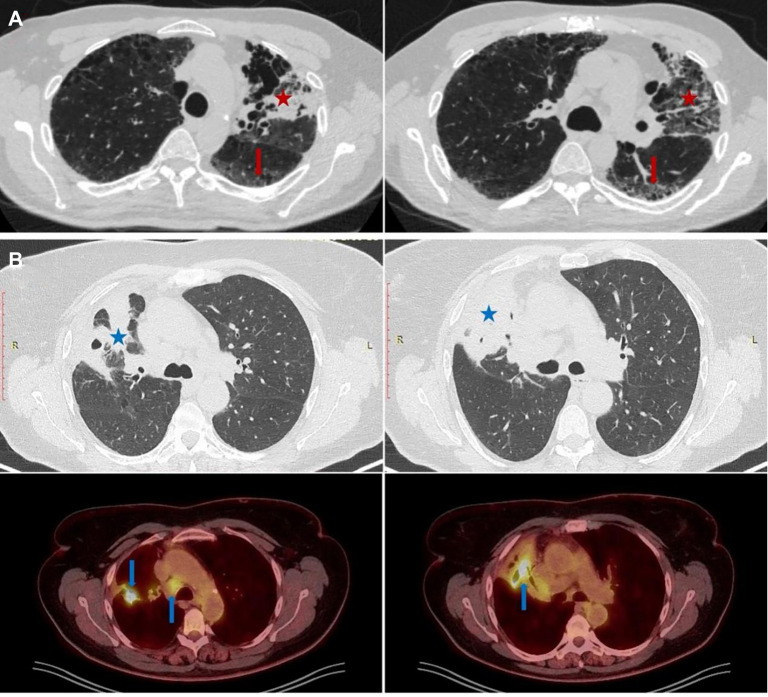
Typical radiological findings from chest CT and PET–CT in cases of pulmonary actinomycosis, as demonstrated in Cases 1 and 2. (A) Case 1. Chest CT revealed a large consolidation with extensive cavitation in the left upper lung lobe (red asterisks), accompanied by usual interstitial pneumonia characterized by subpleural honeycombing and traction bronchiectasis, predominantly observed in the lower lung lobes (red arrows). (B) Case 2. Chest CT (upper row) displayed extensive consolidation and atelectasis in the right upper and middle lung lobes, along with cavitation and mediastinal lymphadenopathy (blue asterisks). PET–CT (lower row) indicated significant increased 18F-FDG uptake (blue arrows) in the consolidative lesion of the right upper lung lobe (SUV_max_ ═ 10.7) and mediastinal lymph nodes (SUV_max_ ═ 4.1 in the right lower paratracheal, 3.5 in the subcarinal, and 2.9 in the right hilar nodal stations). Abbreviations: ^18^F-FDG, fluorine-18 fluorodeoxyglucose; CT, computed tomography; PET–CT, positron emission tomography–computed tomography; SUVmax, maximum standardised uptake value.
